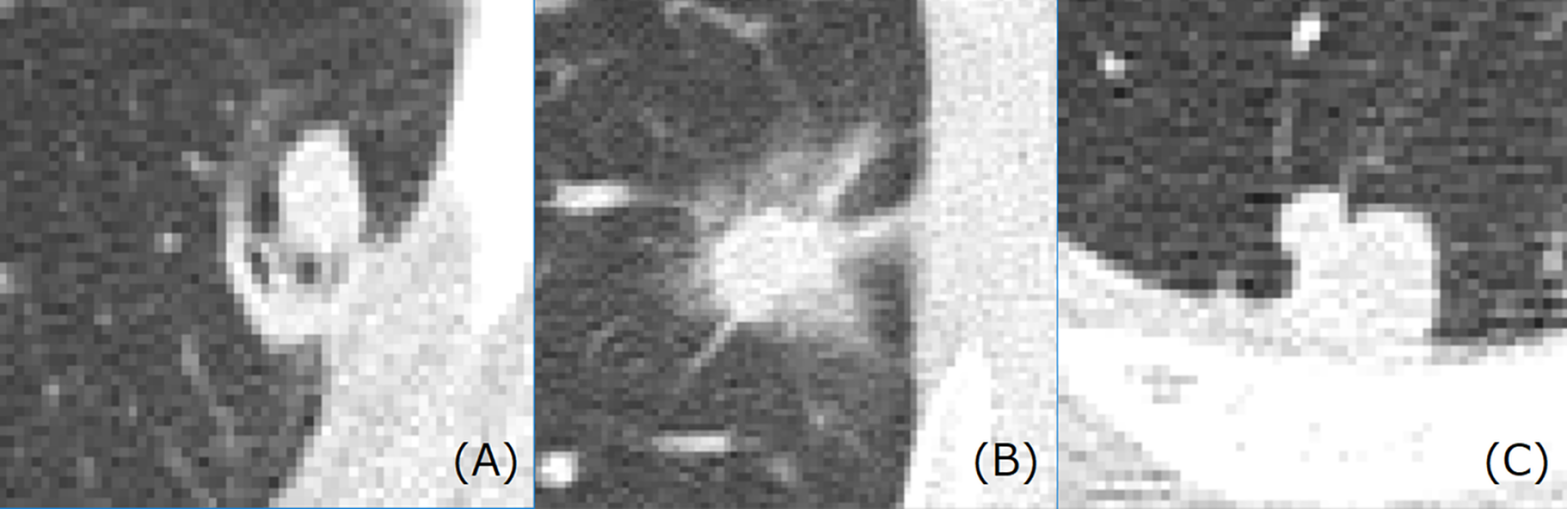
Representative CT images of lung nodules. (A) benign nodule, (B) primary lung cancer and (C) metastatic lung cancer.
